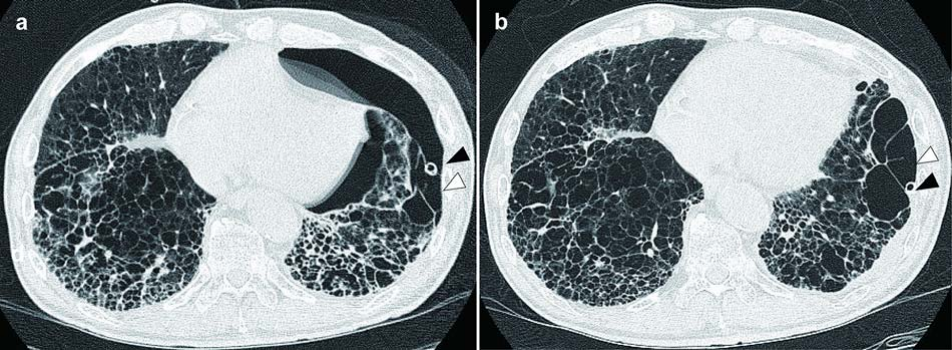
Chest computed tomography images: (a) after placing the second chest tube, the chest tube was placed more precisely over the air leak site; (b) after another attempt at ABPP, air leak had stopped and the left lung was fully inflated. Black arrow: chest tube tip, white arrow: bullae.
